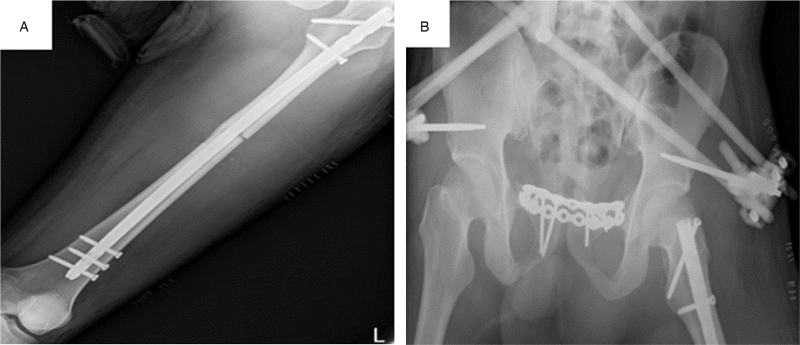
A: Radiograph showing fixation for femoral fractures. B: Radiograph showing fixation for pelvic fractures.
